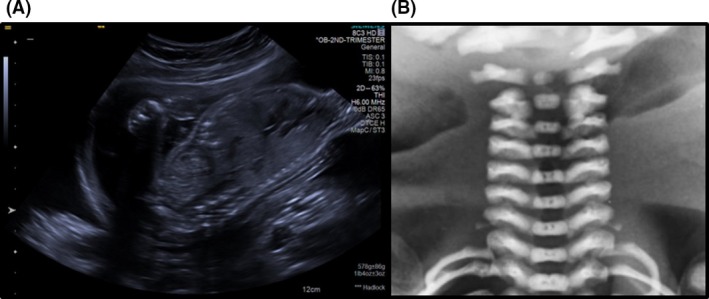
(A) Ultrasound scan image of fetus at 21 weeks showing calcified foci in the upper abdomen (liver). (B) Postmortem X‐ray of the irregularity of the upper cervical vertebrae.
